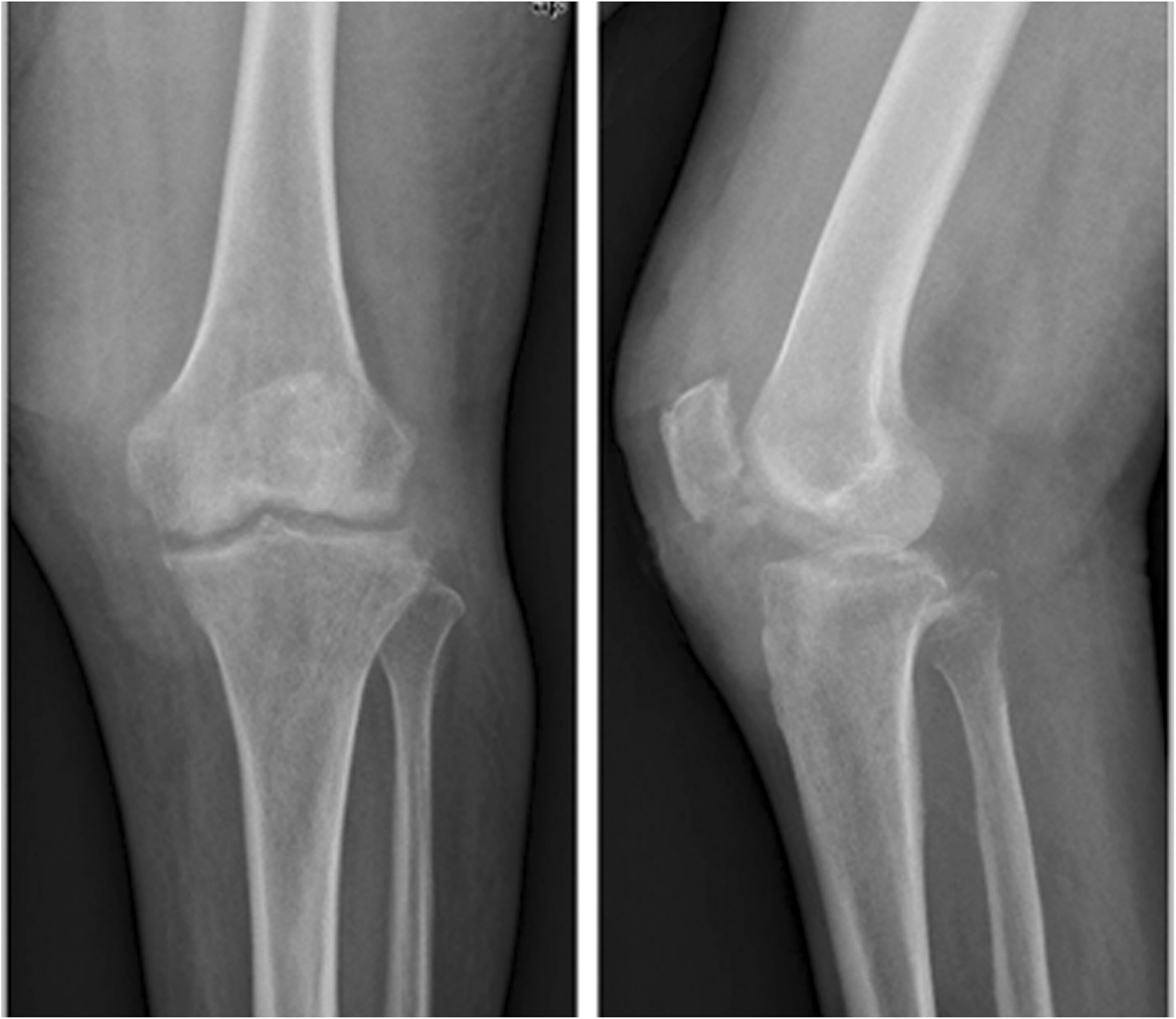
X-ray (anteroposterior and lateral) showing clearly osteoporosis and joint destruction
